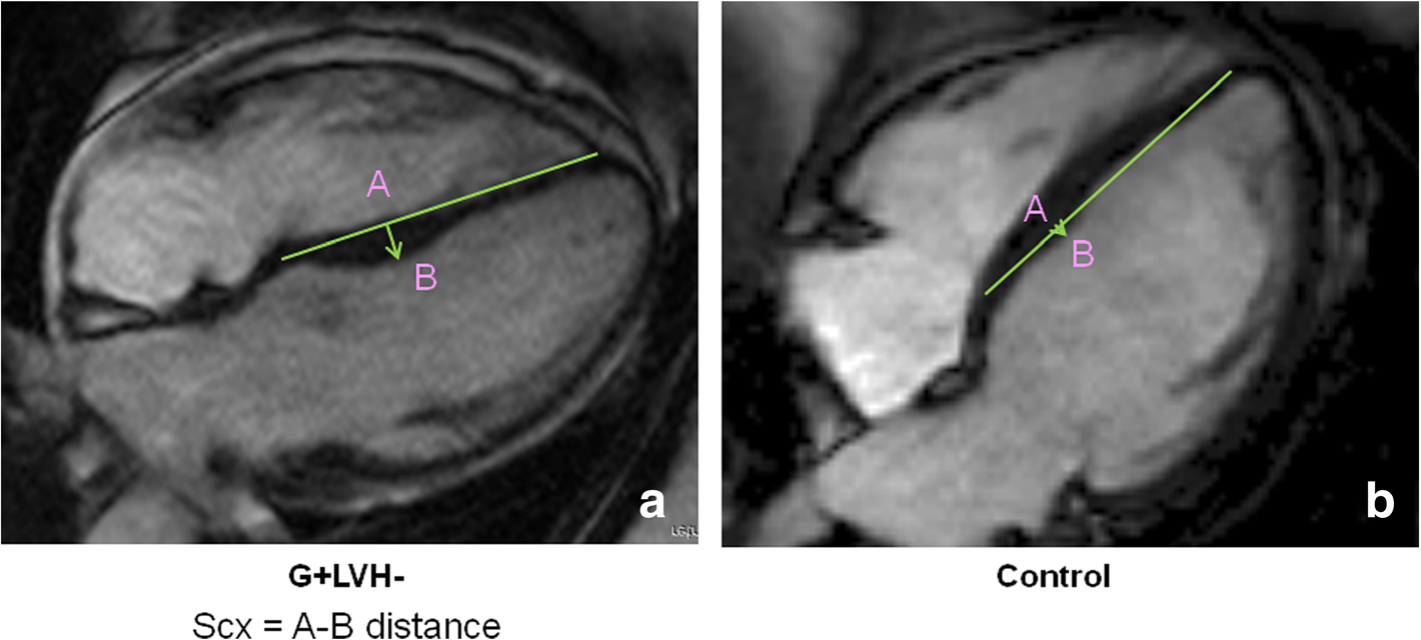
Measurement of septal convexity (SCx) in apical 4 chamber view: performed as the maximal distance (A to B) between the LV endocardial border (B) and the intersection point (A), perpendicularly to a reference line joining at mid-wall the level of tricuspid valve insertion (C) and the apical right ventricular insertion point into the LV (D) in a 49-year old G + LVH- male (a), and in a matched healthy control (b)
